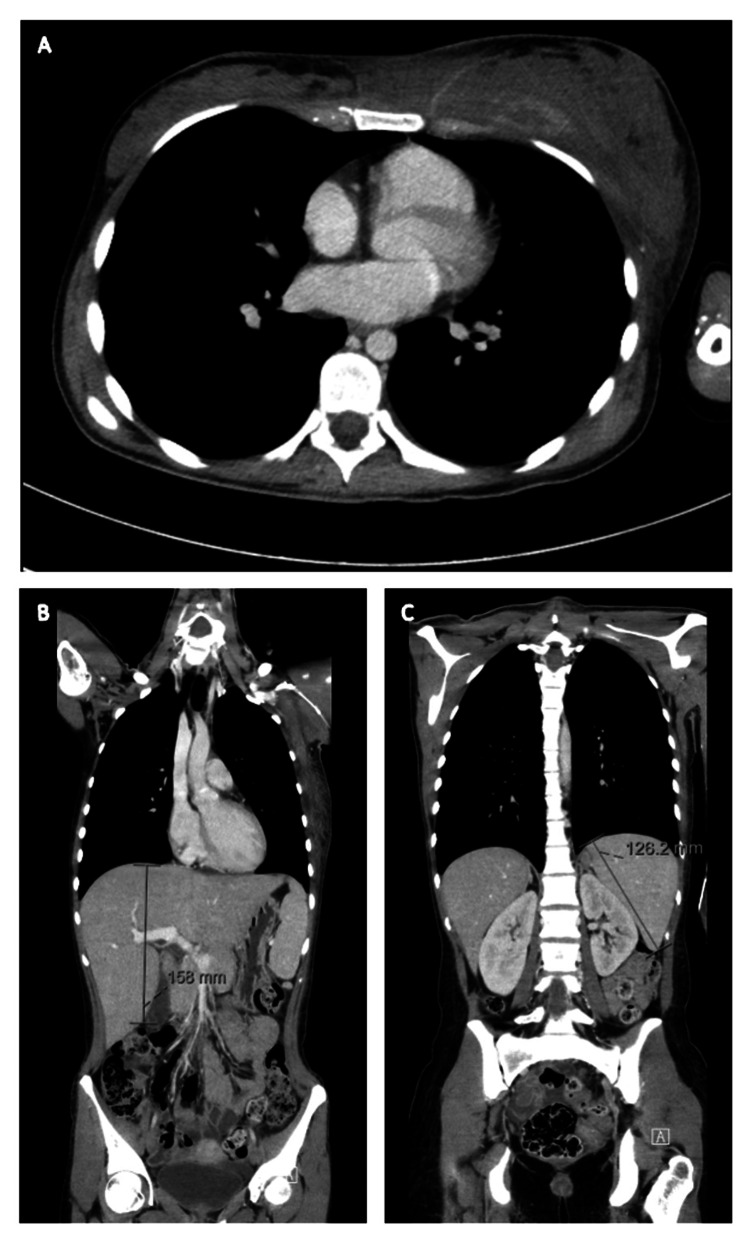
Whole-body computed tomography scan showing a mass with 8 x 3 cm in the deep tissues of the left breast with extension to the pectoralis major muscle (panel A), as well as slight hepatomegaly (panel B) and splenomegaly (panel C).
